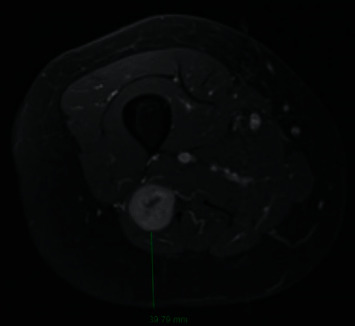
MRI axial showing right sciatic nerve tumor, 4 cm from the skin.
